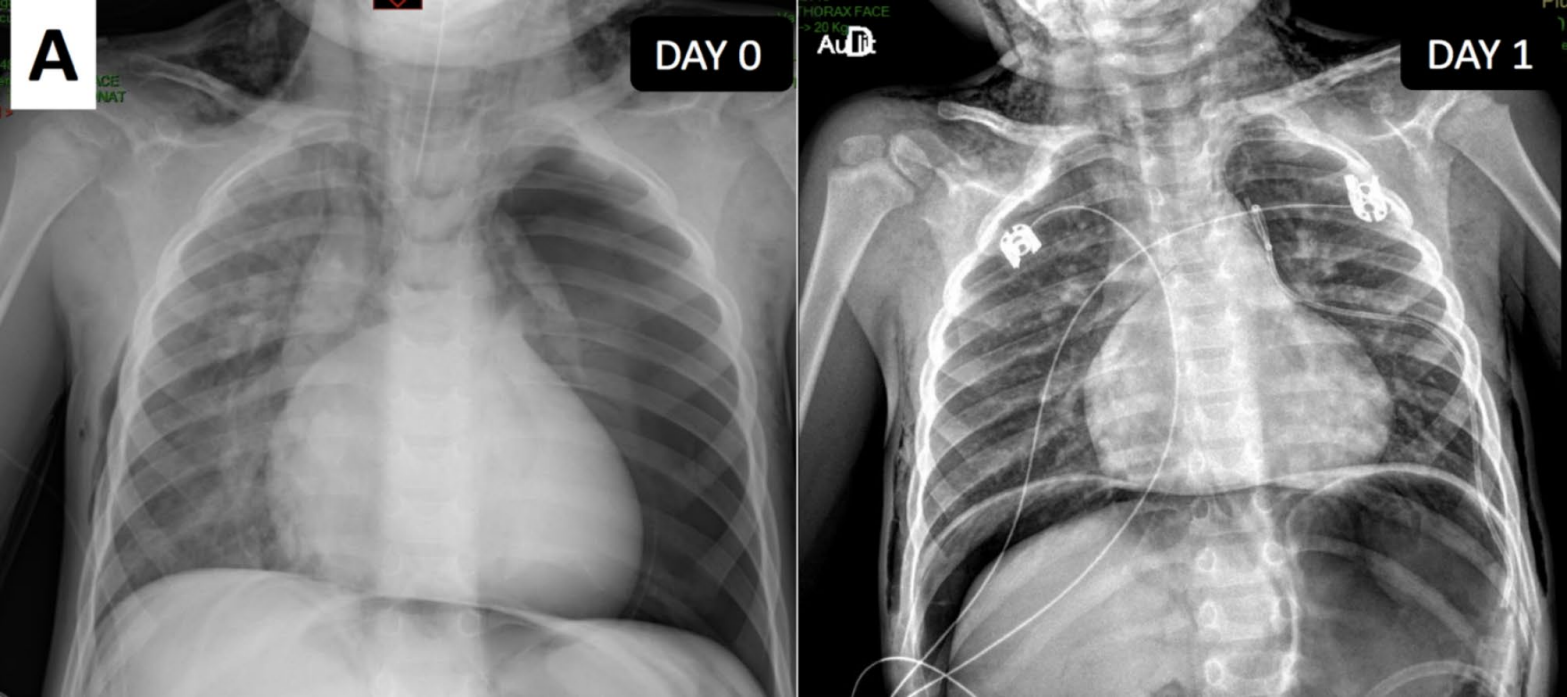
The left thoracic radiograph (Day 0) shows complete left pneumothorax, pneumomediastinum, pneumoperitoneum, and subcutaneous emphysema. On the right radiograph (Day 1), the left lung comes back to its normal position due to the Fuhrman drain. The young patient has been extubated and the major pneumoperitoneum is appreciated
